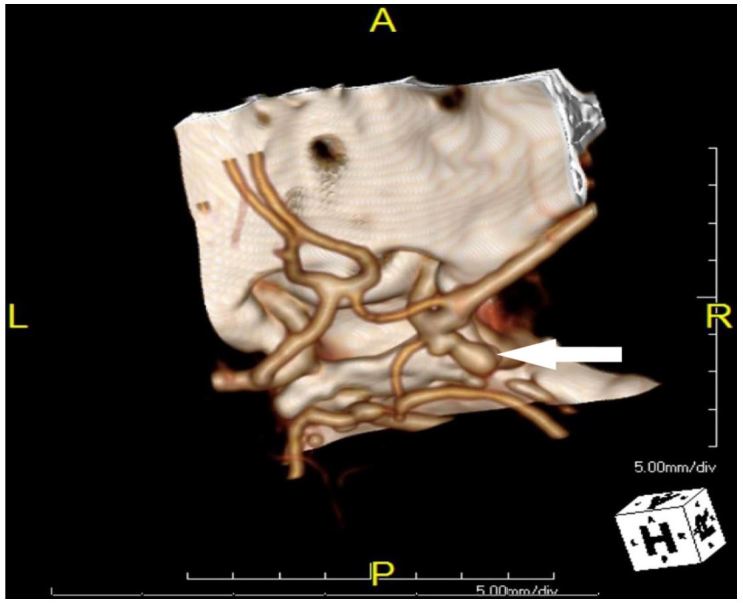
Three-dimensional contrast enhanced computed tomography reconstruction showing bilobed right posterior communicating artery aneurysm.
